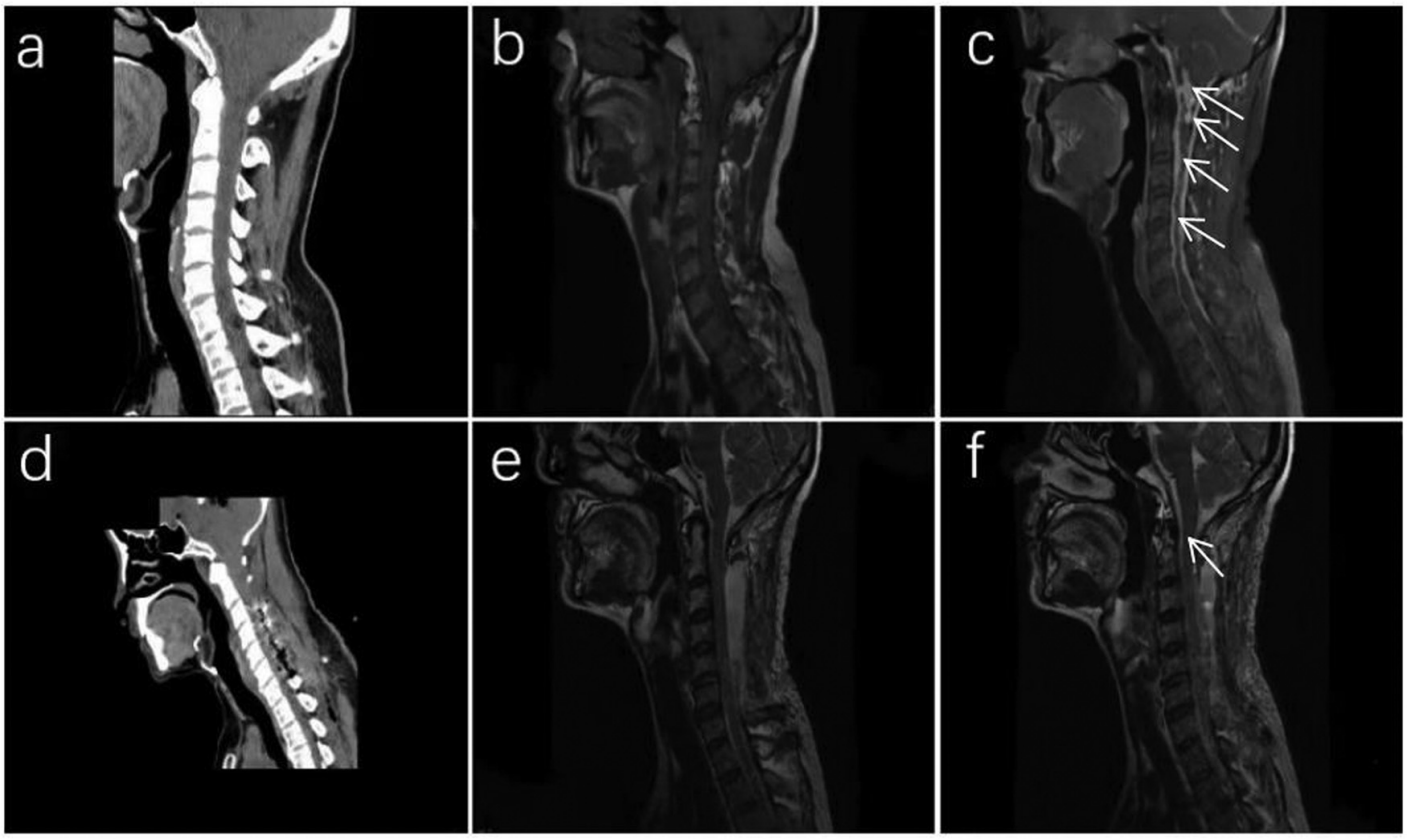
(a–c) Preoperative images of the patient: CT of the head and cervical spine showing no obvious abnormalities (a), MRI showing that the lesion extends from the tentorium cerebella to the seventh cervical spinal canal, enveloping the cervical spinal cord. The lesion is isointense on T1-weighted images and slightly longer range on T2-weighted images, enhanced remarkably and homogeneously after infusion of contrast administration (arrows) (b and c). (d–f) Postoperative images, CT showing changes in the dural lesions after resection (d). Postoperative MRI showing that a part of the thickened dura of the foramen magnum and C1 vertebral body is nodular, while nodular signals in the other regions are significantly reduced (arrows) (e and f).
